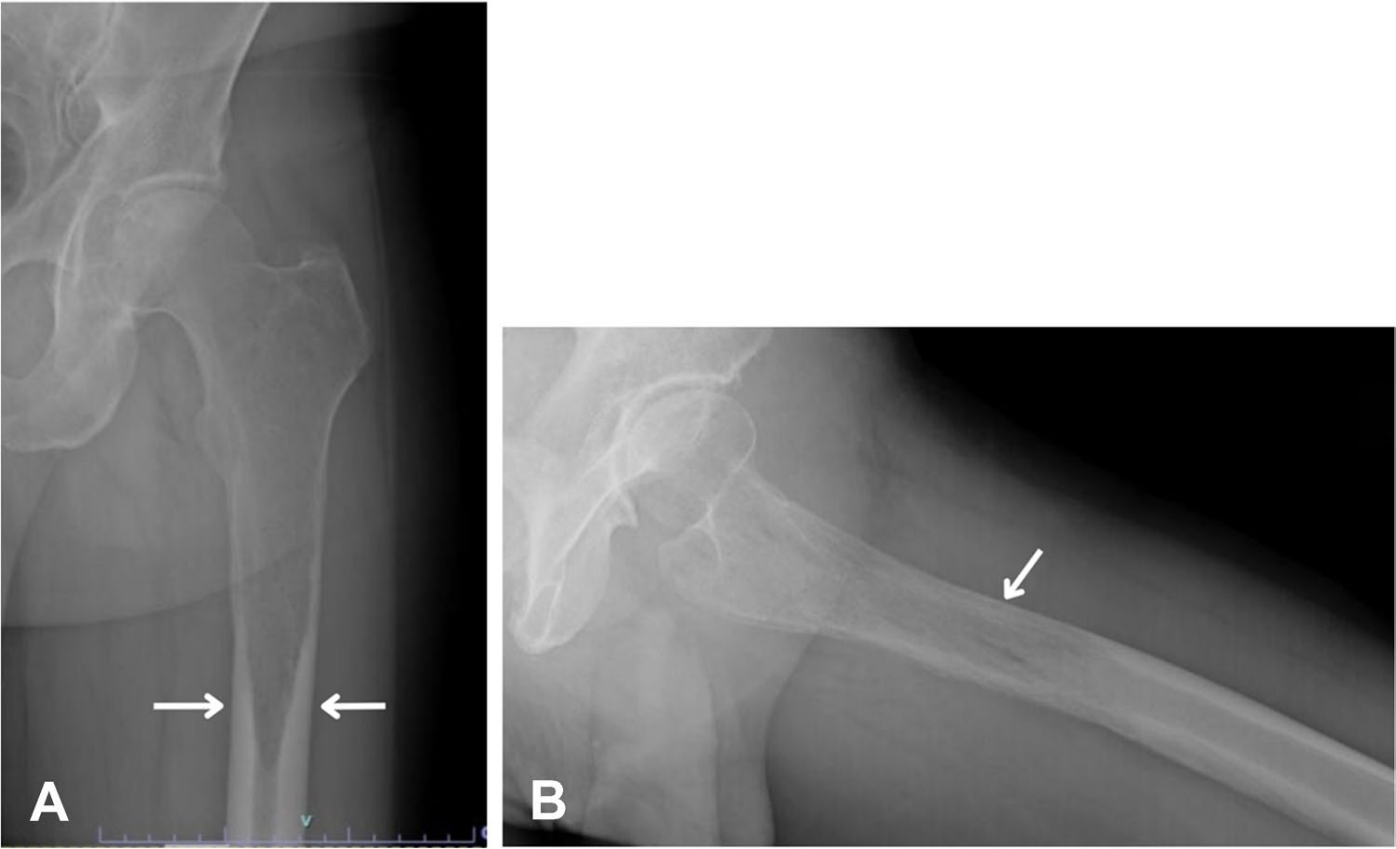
A Anteroposterior radiograph demonstrates a flame shape (blade of grass—arrows) lytic lesion in the proximal diaphysis, initially attributed to the lytic phase of Paget’s disease of bone (PDB). B Lateral radiograph of the proximal femur shows osteolysis, significant endosteal scalloping and coarsened trabeculae also suggesting PDB (arrow)
